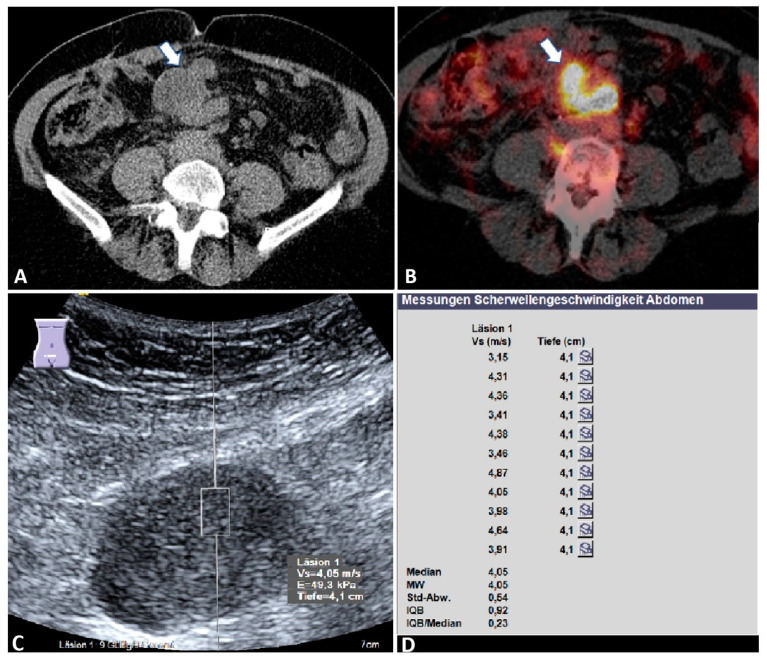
Malignant mesenteric mass. A 76-year-old female patient with a known history of ovarian cancer and suspected recurrence on staging. (A) Computed tomography image (left) shows a hypointense mesenteric mass (arrow) (courtesy of Prof. Dr. Mahnken, Department of Radiology, University Hospital Marburg); (B) positron emission tomography–computed tomography reveals a high intensity of fluorodeoxyglucose uptake within the mass (arrow)), indicating mesenteric metastasis (courtesy of Prof. Dr. Luster, Department of Nuclear Medicine, University Hospital Marburg); (C) B-mode ultrasound showing a hypoechoic mesenteric mass; (D) the final acoustic radiation force impulse (ARFI) report of the same mass, showing a mean ARFI velocity (MW) of 4.05 m/s. Läsion 1: lesion 1; Vs (m/s): velocity in meter per second; Tiefe (cm): depth in centimeter; MW = mean value (Mittelwert); Std-Abw.: standard deviation (Standard Abweichung); IQB = interquartile range (Interquartilbereich); E: elasticity in Kilopaskal (kPa).
